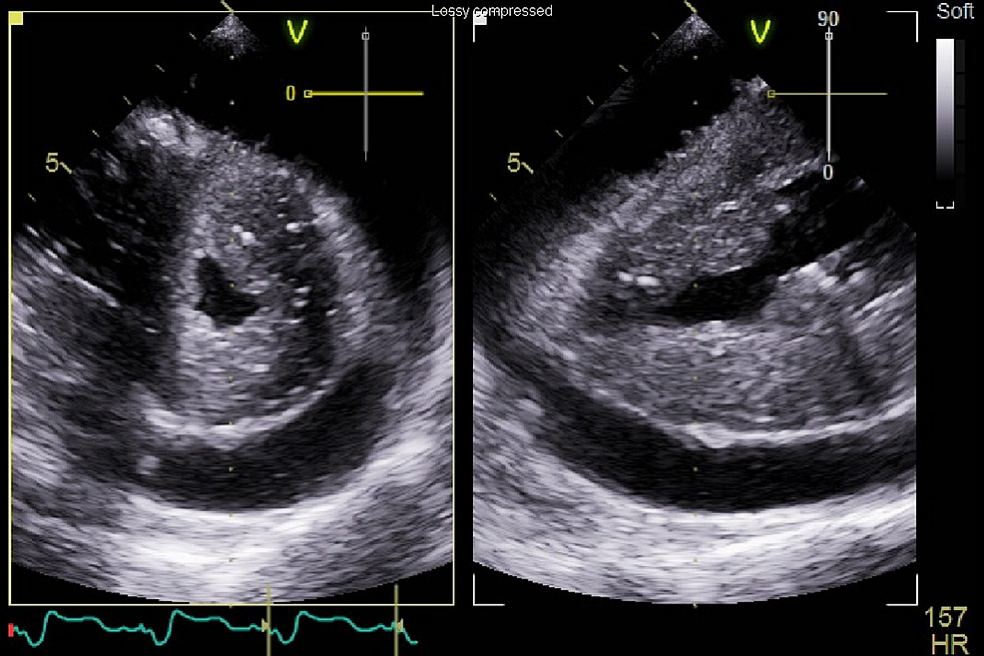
Preoperative transesophageal echocardiogram shows large circumferential pericardial effusion in transgastric short axis (left) and long-axis (right) views.
